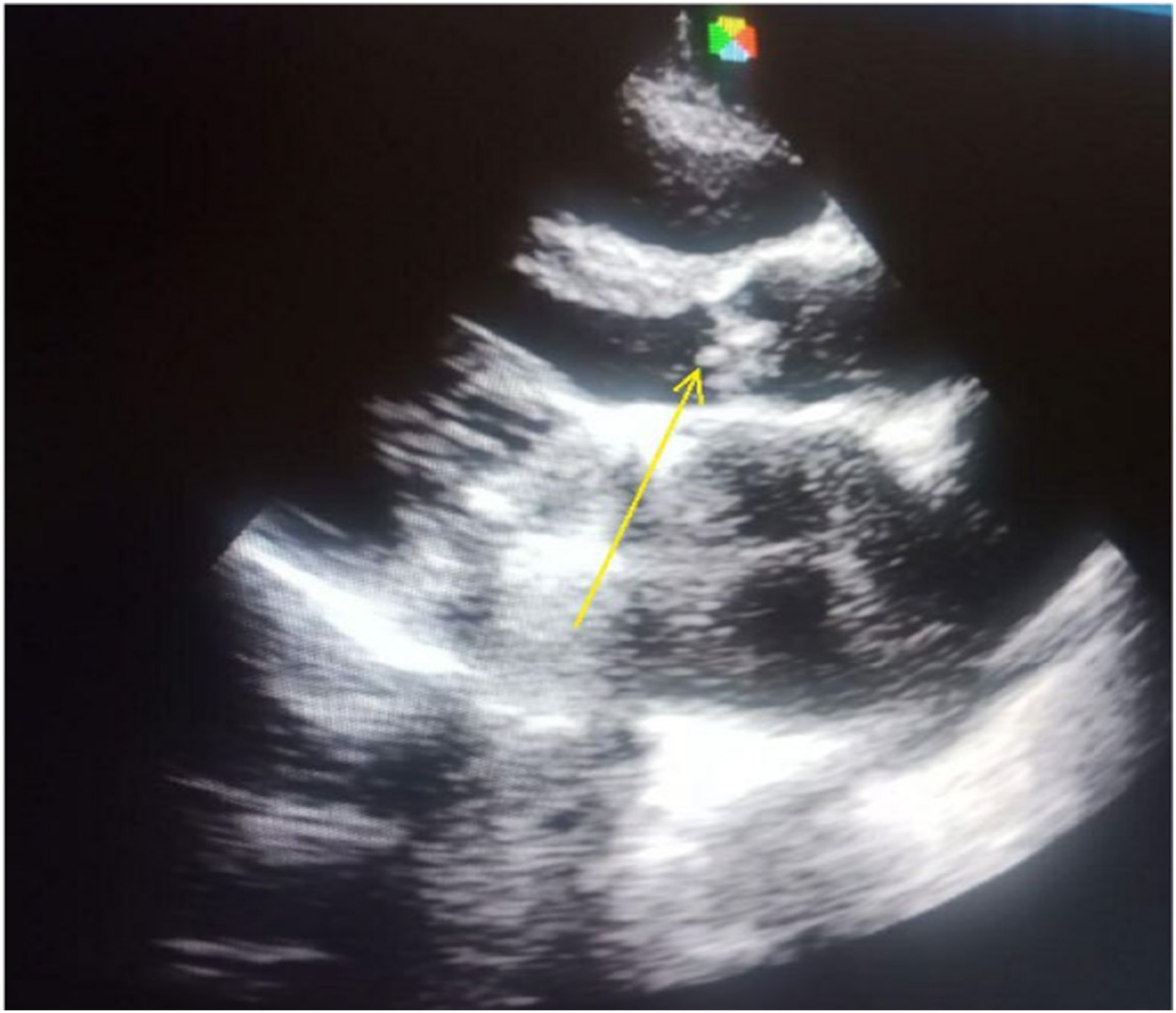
Echocardiography image post‐treatment: arrow head shows aortic valve vegetation (the prosthetic mitral valve vegetation has disappeared).
